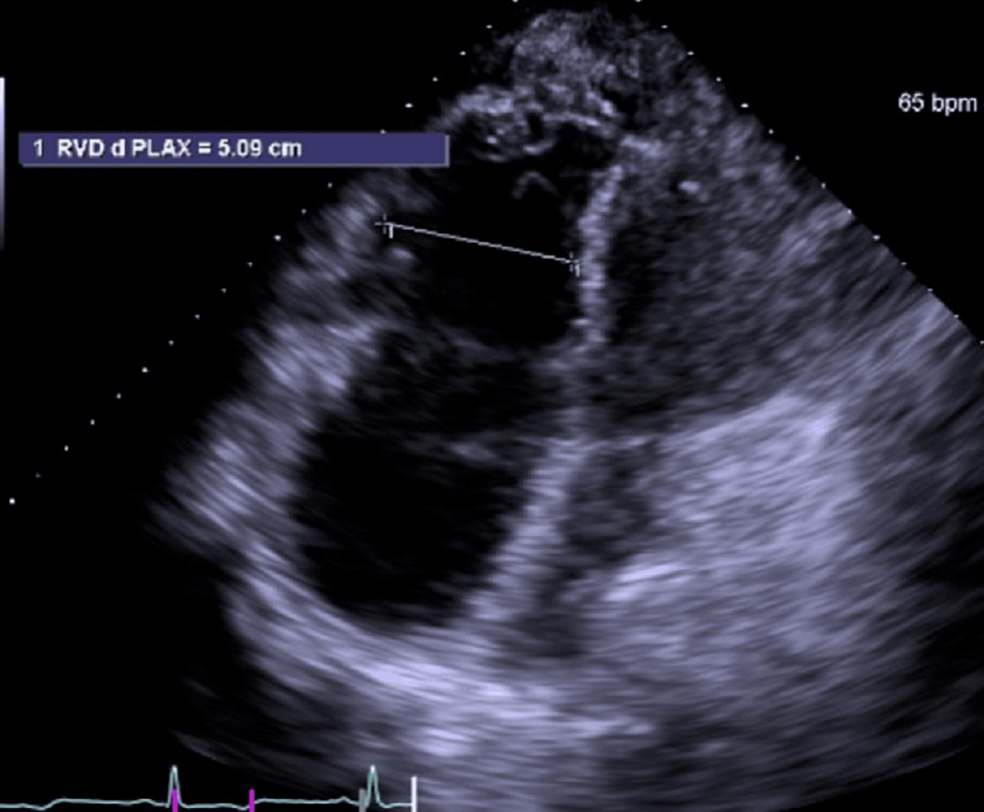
Preprocedure echocardiogram. RVD, right ventricle diameter
